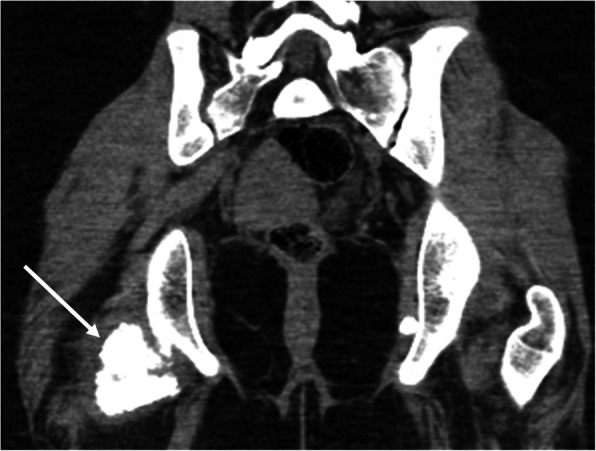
Coronal CT image of the pelvis from the same examination as Fig. 1 again shows the large area of soft tissue calcification in the right ischiofemoral space (white arrow)
